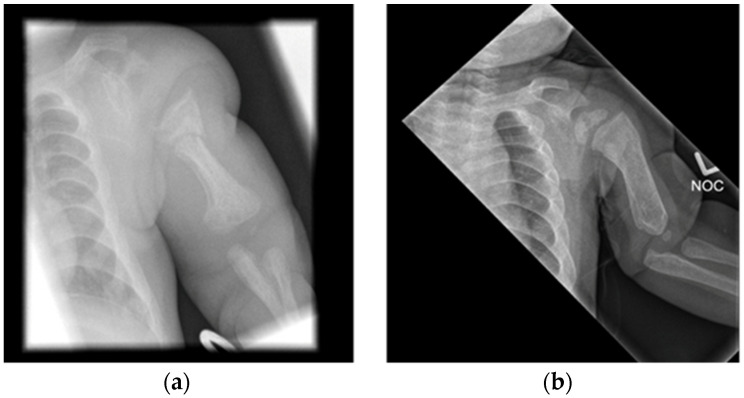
Acute fracture with healing at 4 months. Patient 4 (a) radiograph of left humerus on day 1 shows a transverse fracture of the left humerus. (b) Follow-up radiograph 4 months later showing interval healing of the fracture and resolution of the periosteal cloaking. There are already emerging features of dysostosis multi-plex with widening of the shaft and short length of the humerus and coarse trabecular markings as well as thickening of the ribs.
